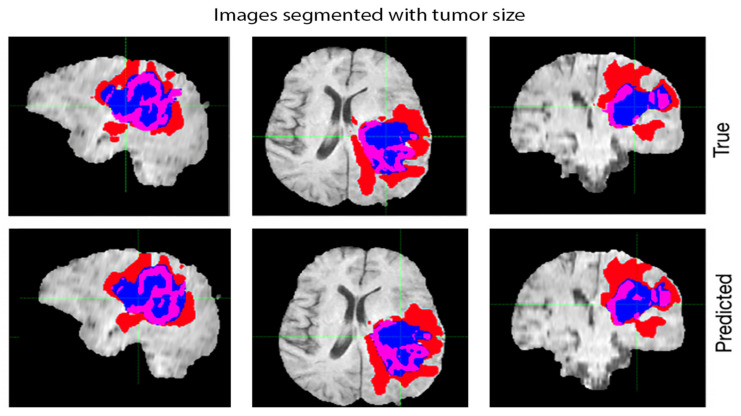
Segmentation of Brain Tumors using MRI Scans: A Comparative Analysis of Three Image Processing Techniques.
